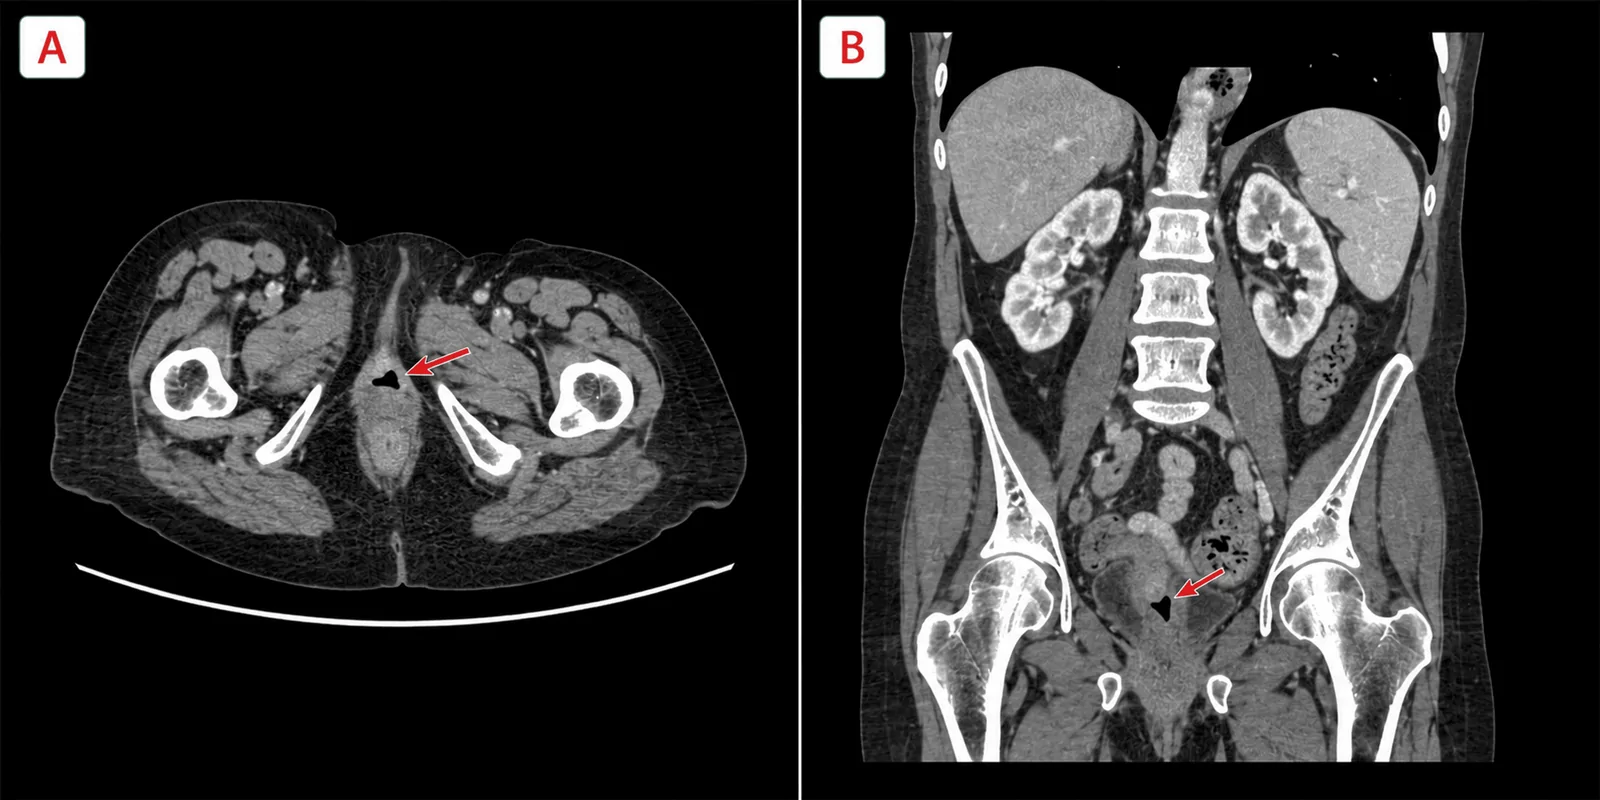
CT abdomen and pelvis CT demonstrating air within the vaginal vault (red arrows) on axial (A) and coronal (B) images, consistent with rectovaginal fistula formation, obtained one month after symptom onset CT: computed tomography
